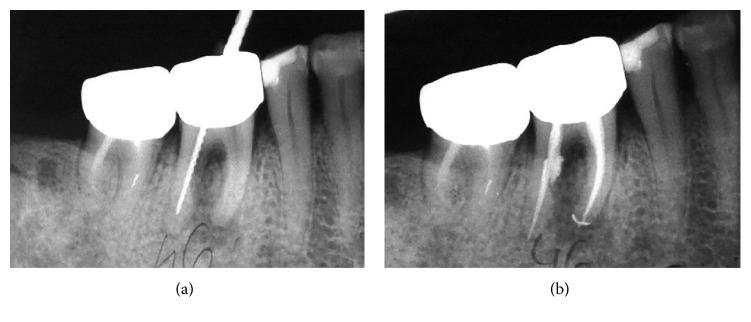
(a) The endodontic lesion extended from the apex of the mesial root of tooth 4.6. towards the furcation dome, causing an external resorption of the mesial aspect of the distal root. (b) The regular shaping with nickel-titanium (Ni-Ti) instruments of the distal canal resulted in stripping with extrusion of the filling material, which became obvious only on postoperative radiographic examination.
